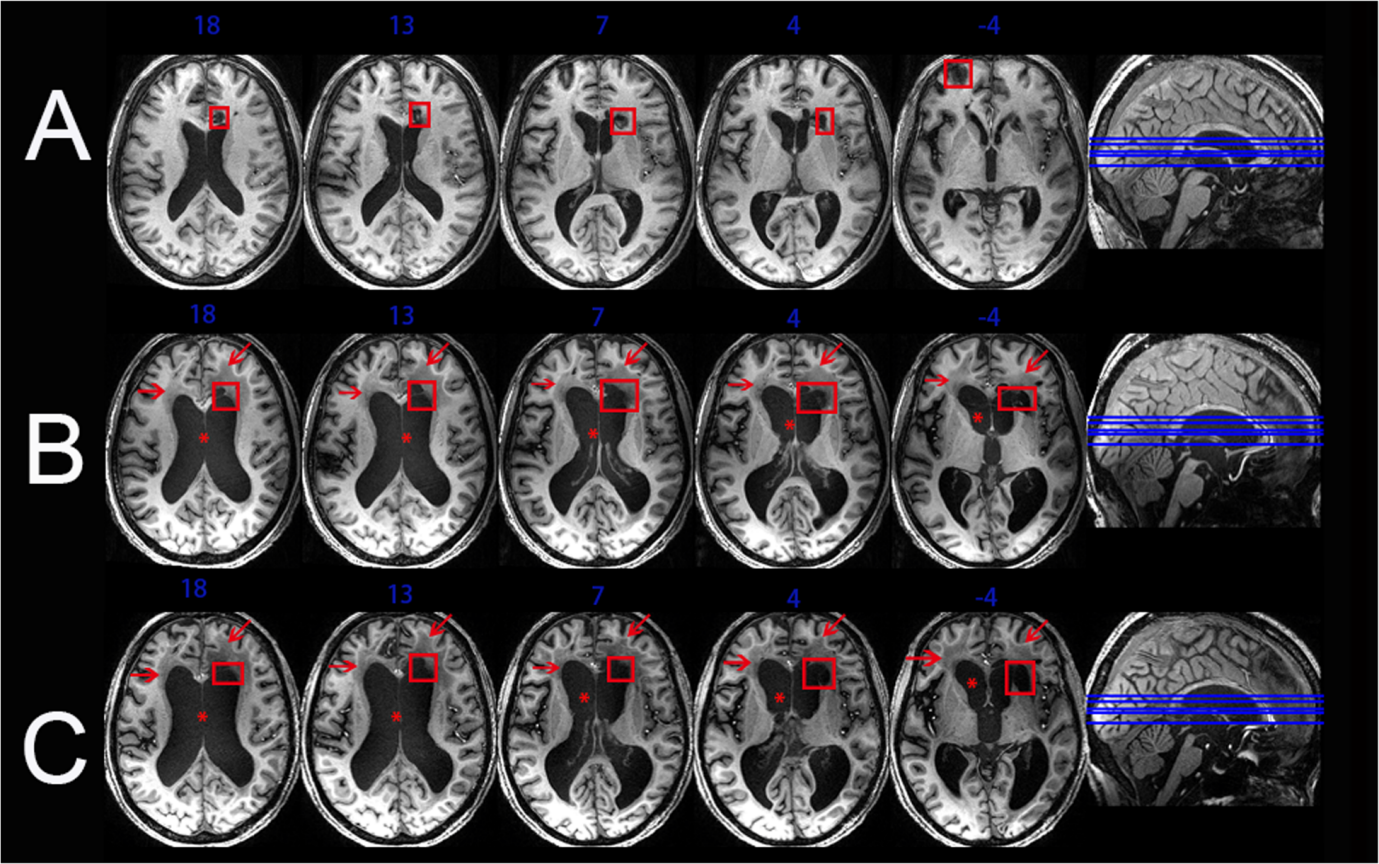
Magnetic resonance imaging (MRI) depicting macroscopic structural changes in the brain. The normalized T1-weighted axial sections of an entire MRI image obtained at (a) 1.5 months (b) 7.5 months and (c) 17.5 months after initial injury. Red boxes indicate the focal malacia; Red arrows indicate the oedema and/or gliosis area; Red * indicate the enlarged bilateral ventricles
